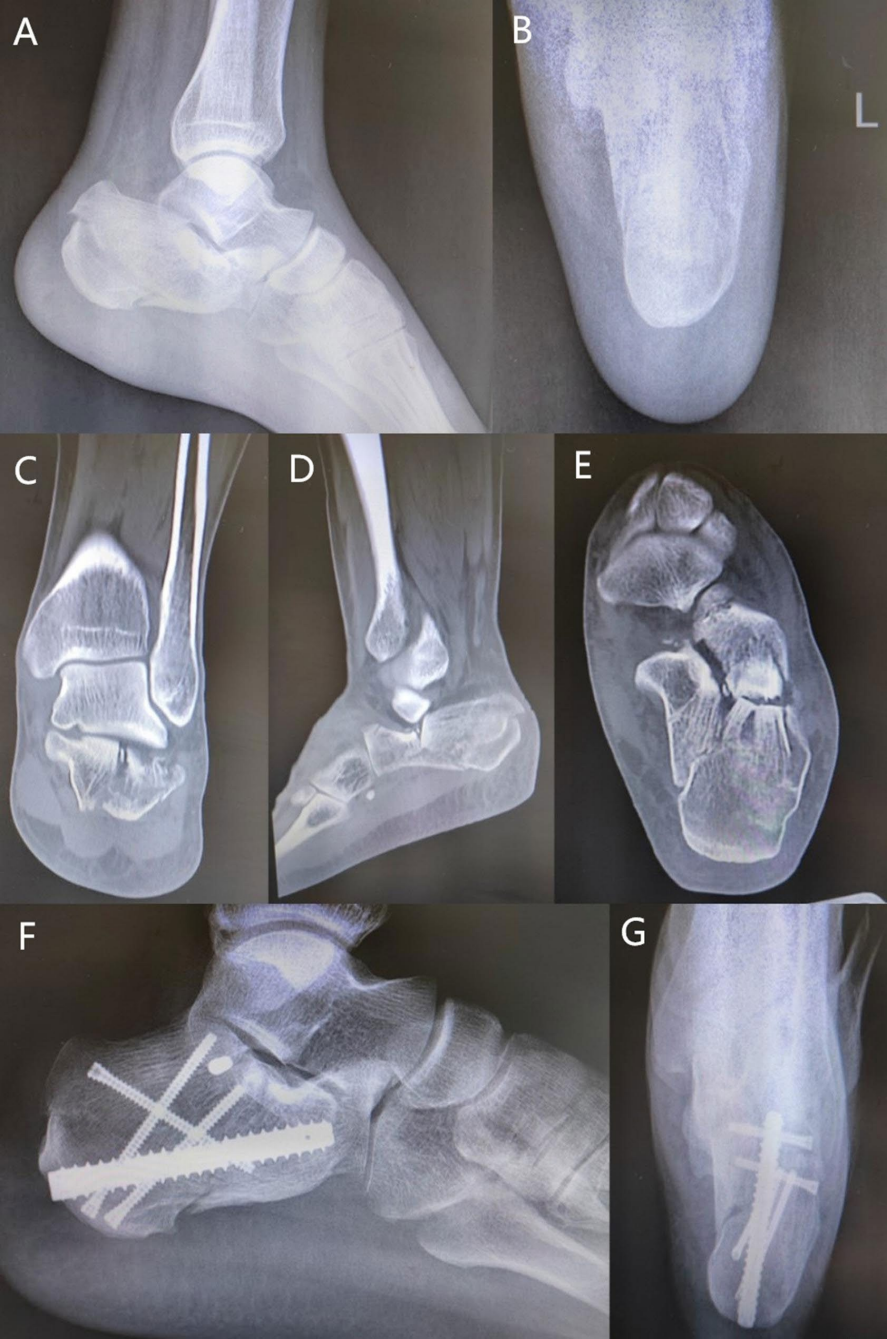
Case 2. Male, 43 years old, who fell from a roof X-ray showed a calcaneous fracture. Later view (a) Axial view (b).
